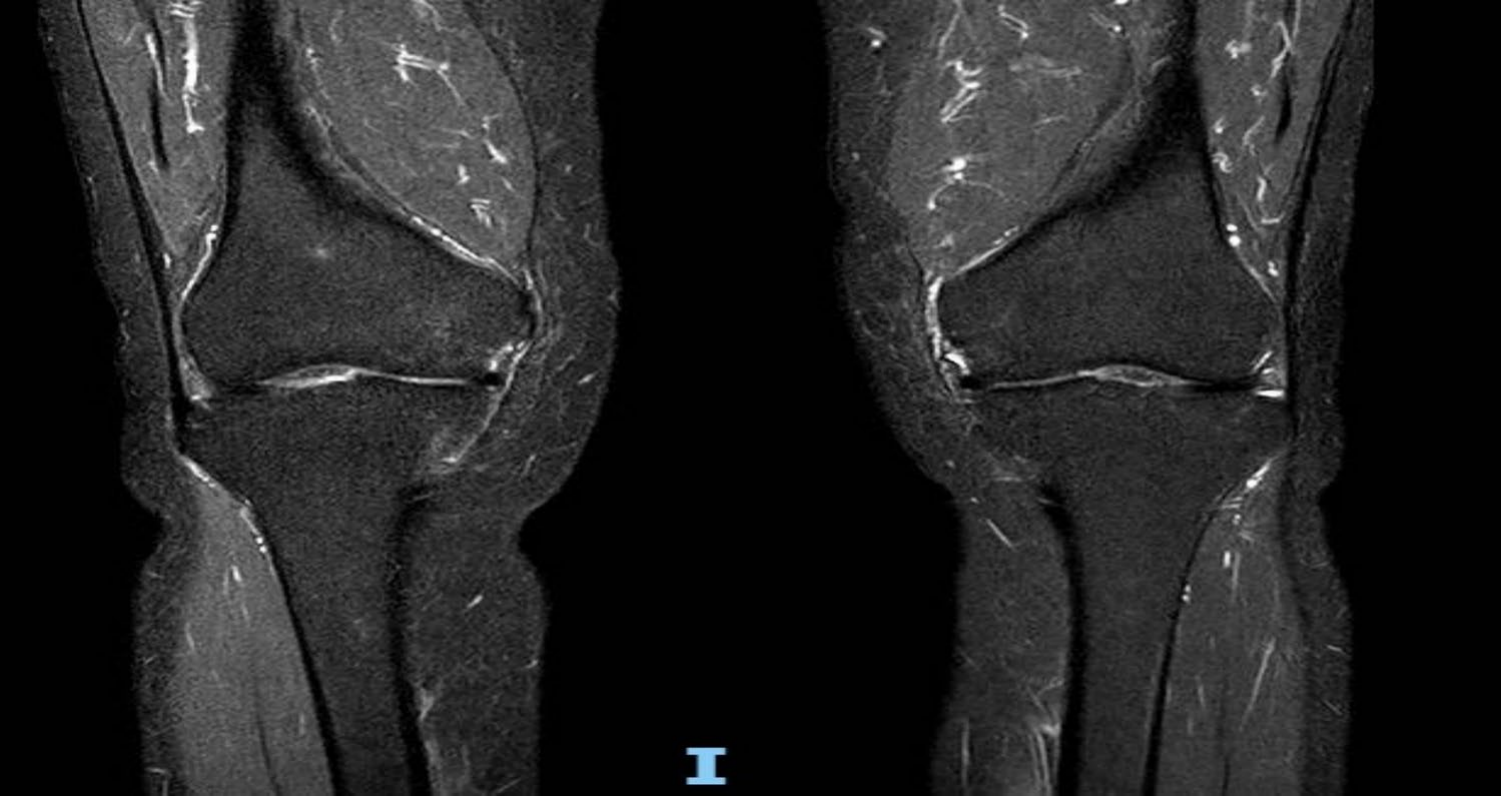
Bilateral knee MRI, nine months post-transplant and five months of native vitamin D supplementation initiation
